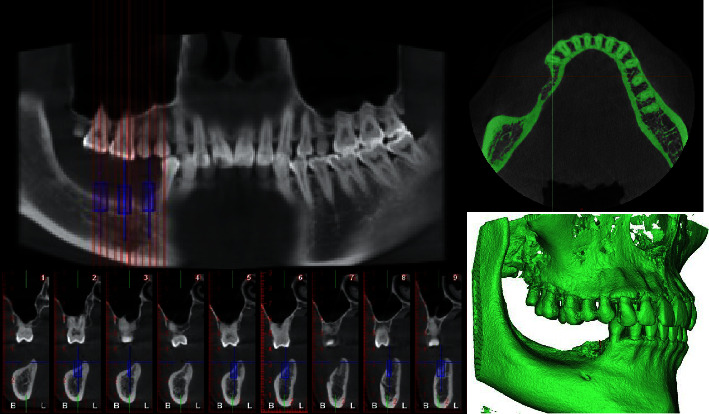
CBCT screenshot and 3D reconstruction of severe bone defects in the posterior mandibular area.
